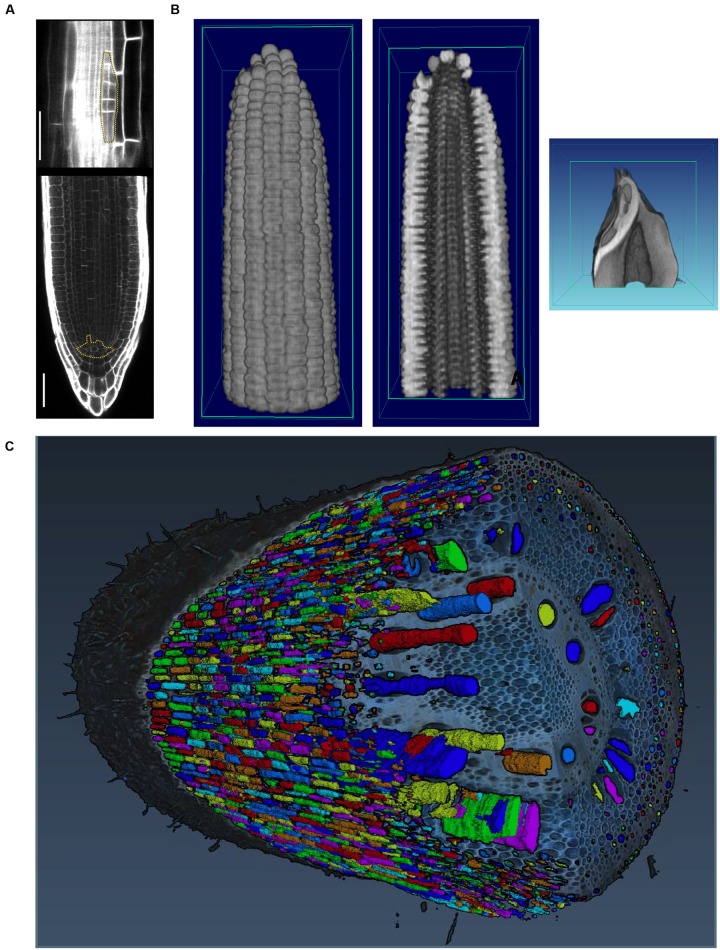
Imaging techniques to capture plant morphology. (A) Confocal sections of an Arabidopsis root. The upper panel shows a new lateral root primordium at an early stage of development (highlighted in yellow). At regular intervals new roots branch from the primary root. The lower panel shows the primary root meristem and the stem cell niche (highlighted in yellow) from which all cells derive. Scale bars: 100 μm. Images provided by AM (Heidelberg University). (B) Computational tomographic (CT) x-ray sections through a reconstructed maize ear (left and middle) and kernel (right). Images provided by CT (Donald Danforth Plant Science Center). (C) Laser ablation tomography (LAT) image of a nodal root from a mature, field-grown maize plant, with color segmentation showing definition of cortical cells, aerenchyma lacunae, and metaxylem vessels. Image by Jennifer T. Yang and provided by JPL (Pennsylvania State University).
